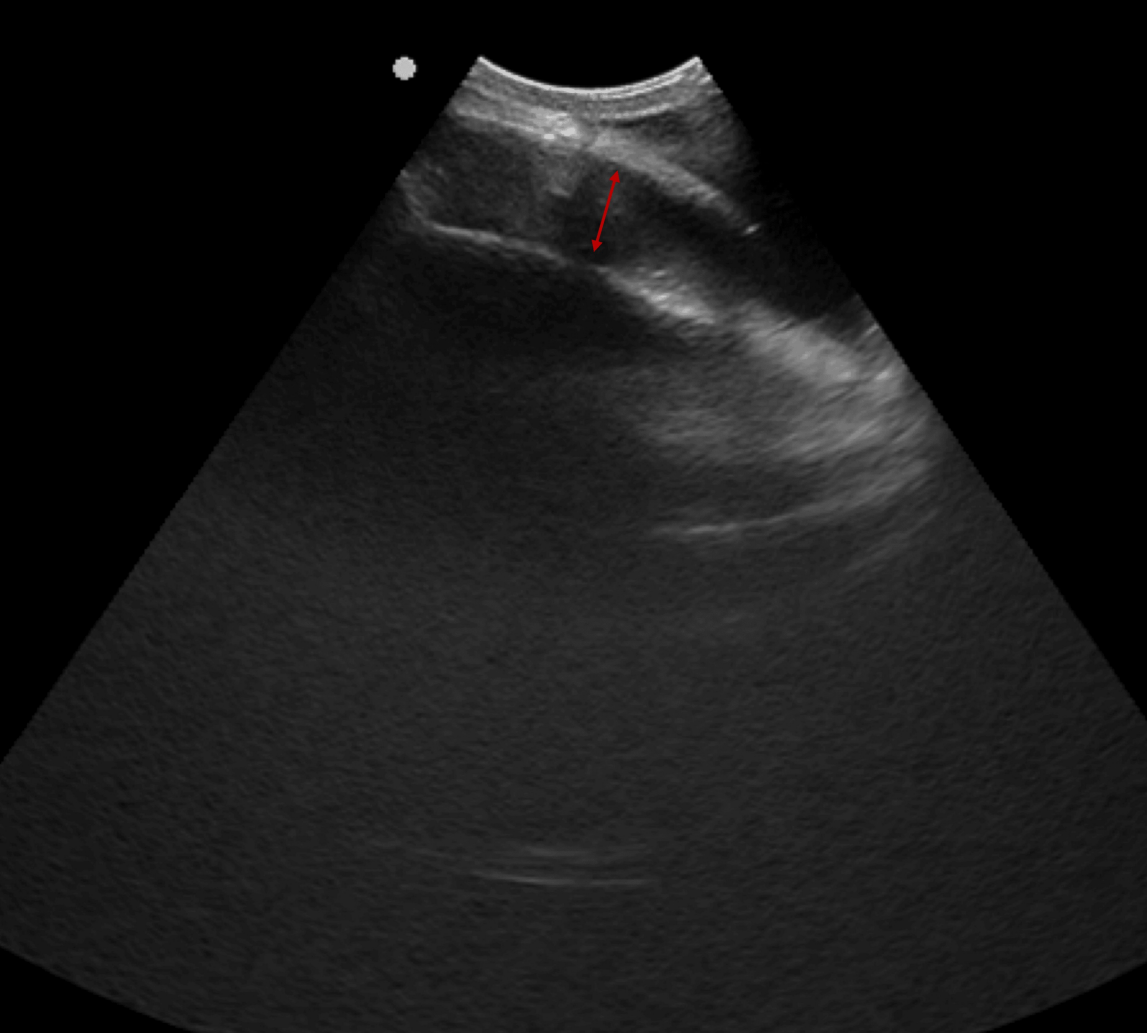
Cranial ultrasound -a small anechoic collection is identified, with simple ultrasound characteristics, not organized, in a subaponeurotic location. Red arrow: highlights the collection area.
